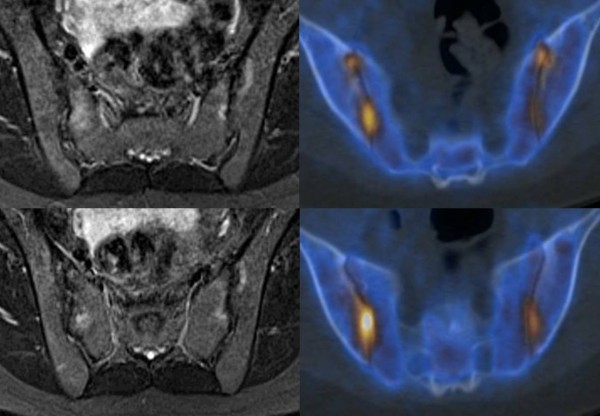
Thirty-year-old female patient (patient number 5). Patient with positive findings in all four inferior iliosacral quadrants both on MRI and F PET/CT without exact overlap. Axial STIR images (left) and fused PET/CT images (right).
